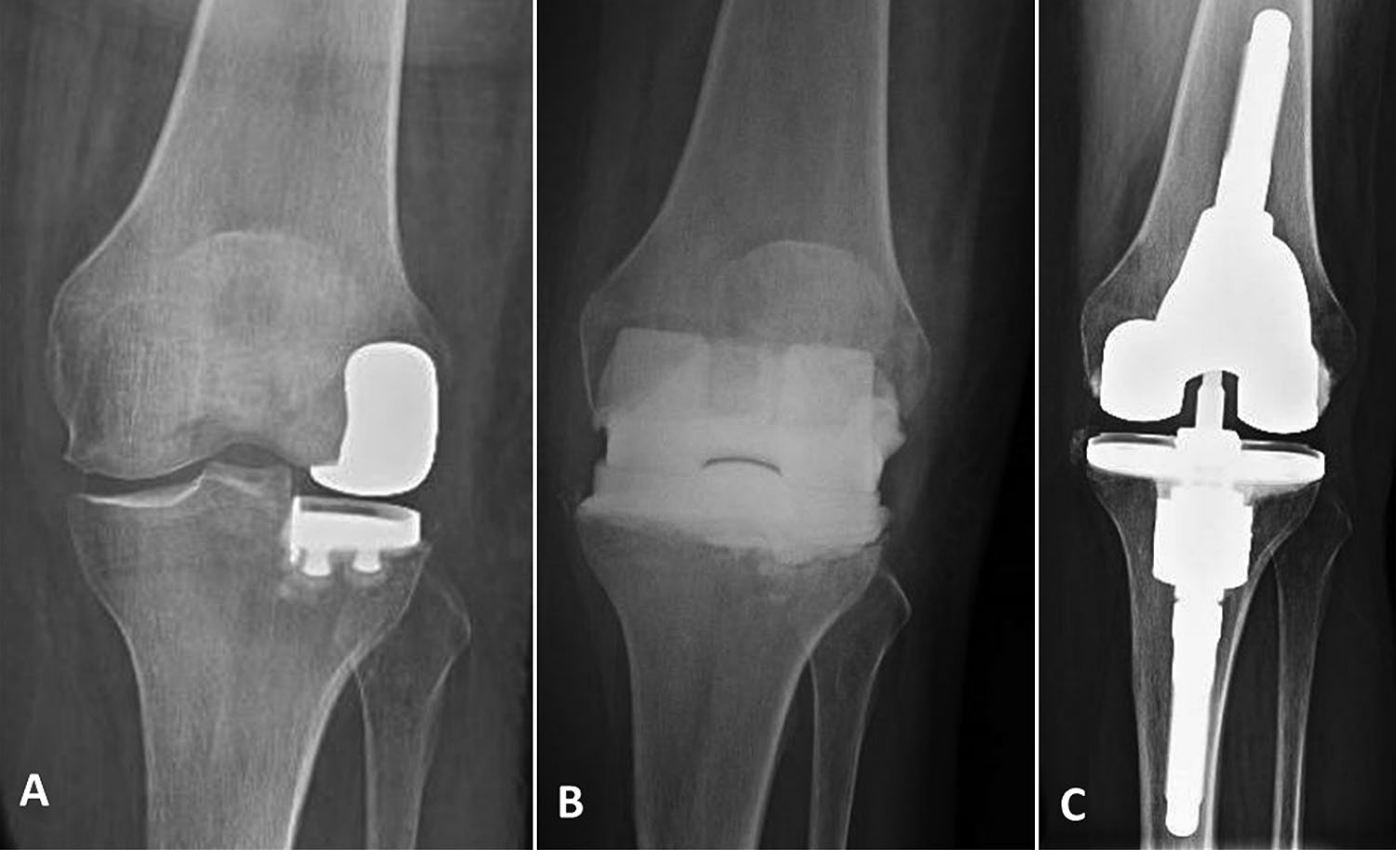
Radiological analysis of a patient affected by left UKA PJI. A Preoperative X-ray showing lateral left UKA. B Articulating spacer. C 3-year follow-up with optimal implant alignment and firm osseointegration of the revision prosthesis
